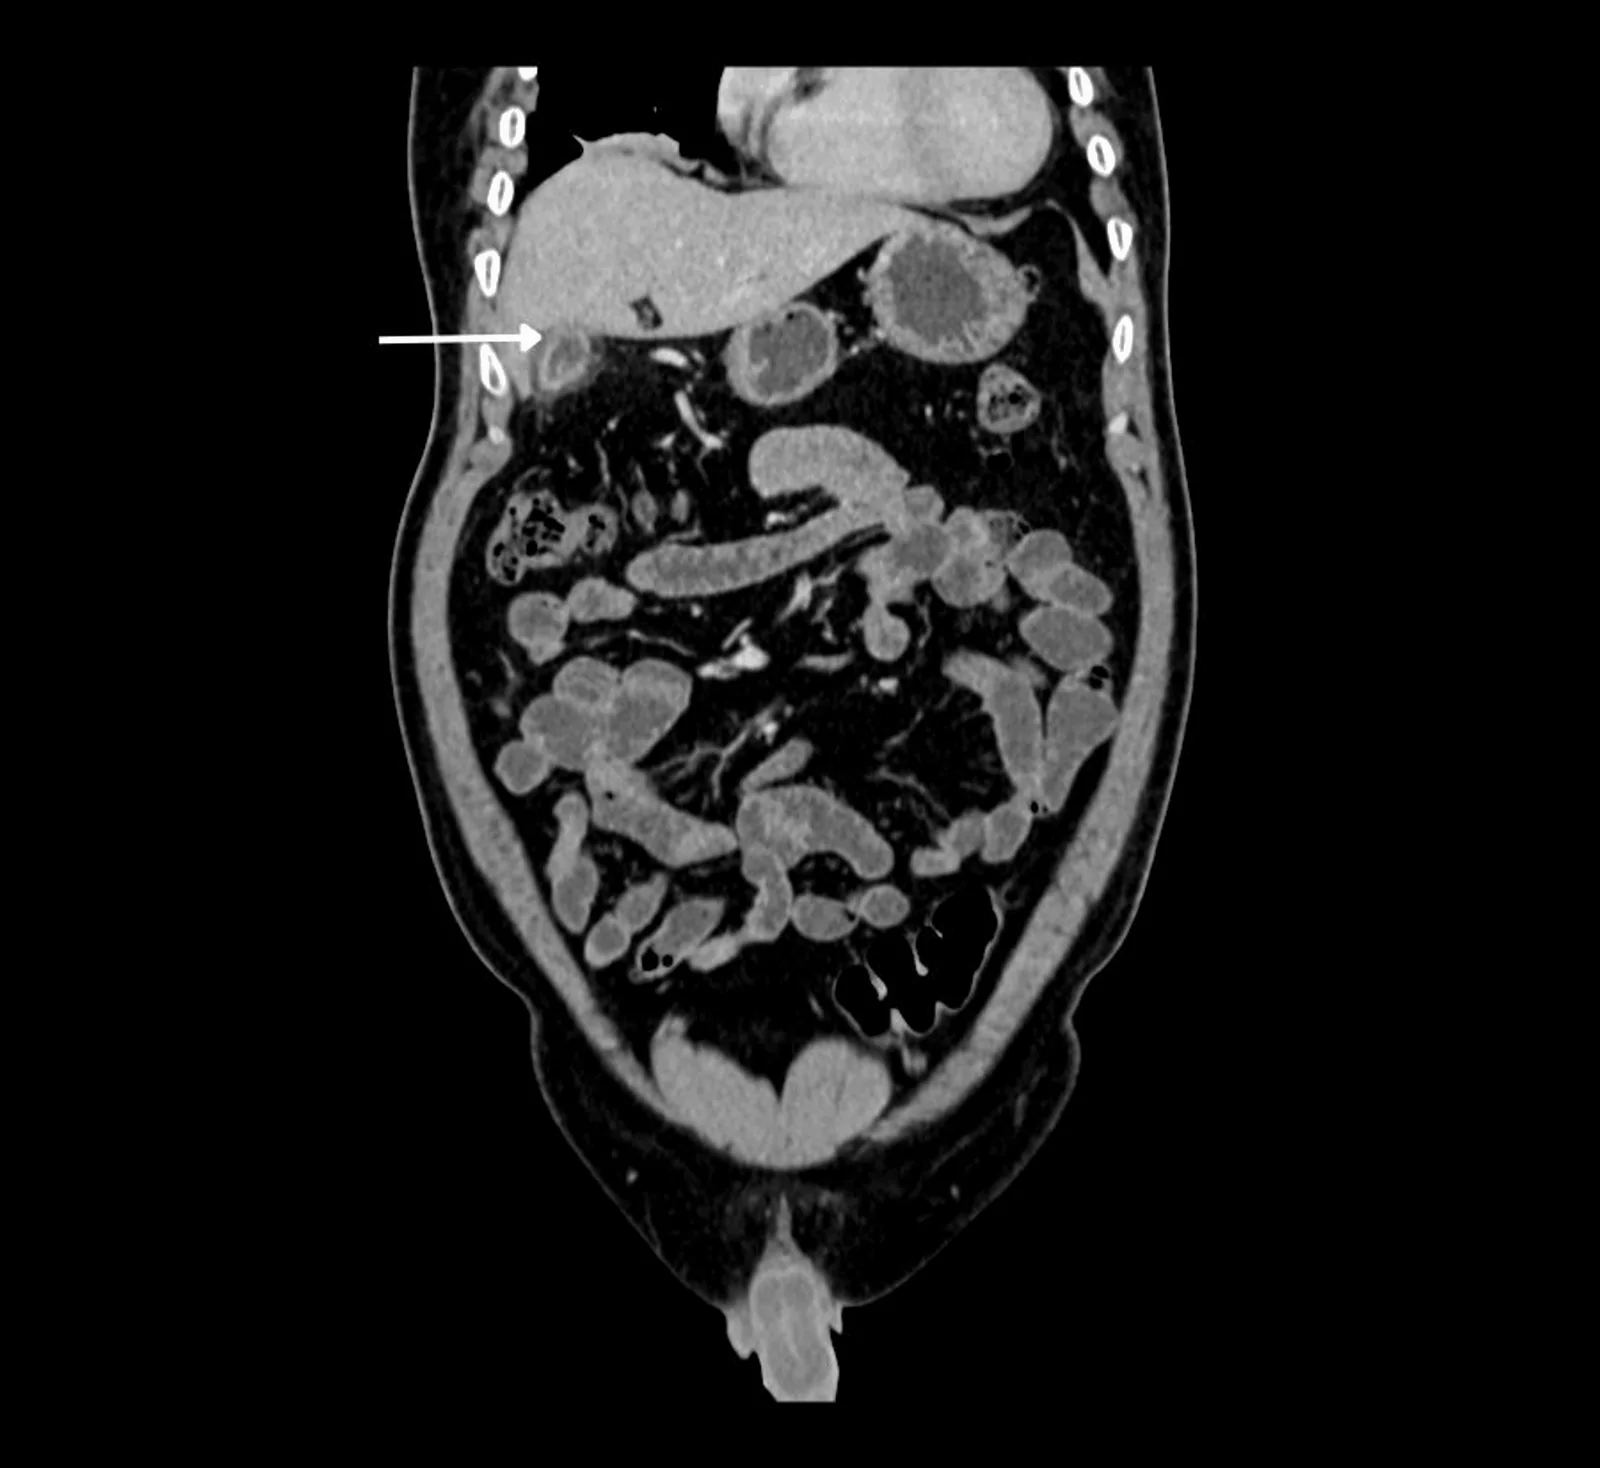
Coronal section of the contrast-enhanced computed tomography (CECT) abdomen showing a thick-walled gallbladder with cholelithiasis The arrowhead points to the gallbladder wall thickening with cholelithiasis.
